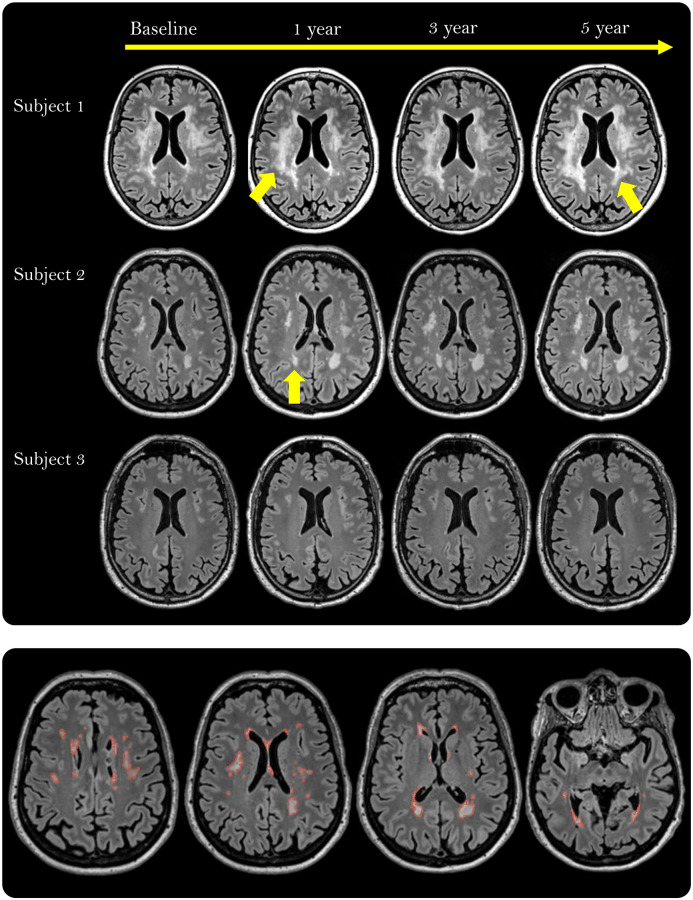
White matter hyperintensities (WMHs) depicted as white, well-defined lesions or confluent areas in white matter on fluid-attenuated inversion recovery (FLAIR) scans in three participants during the 5-year intervention period. The yellow arrows point to areas with growing WMH lesions (Subject 1) or a new lesion (Subject 2). The lower row shows the manually delineated WMHs (red outline) across 4 out of 176 slices in one participant.
